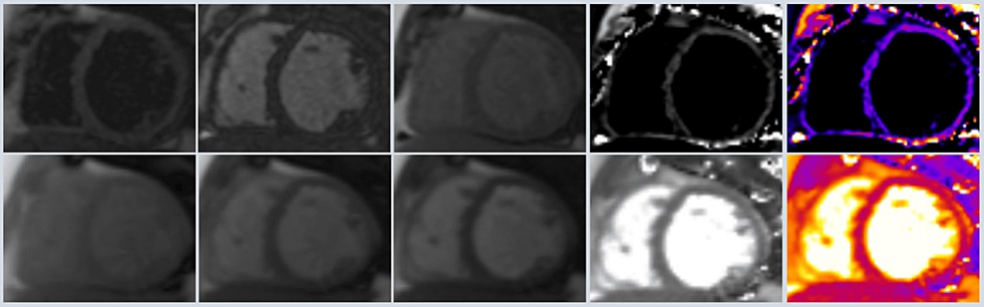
Cardiac MRI The upper and lower panels represent T1 and T2 tissue mapping, respectively, without identifiable abnormalities.
